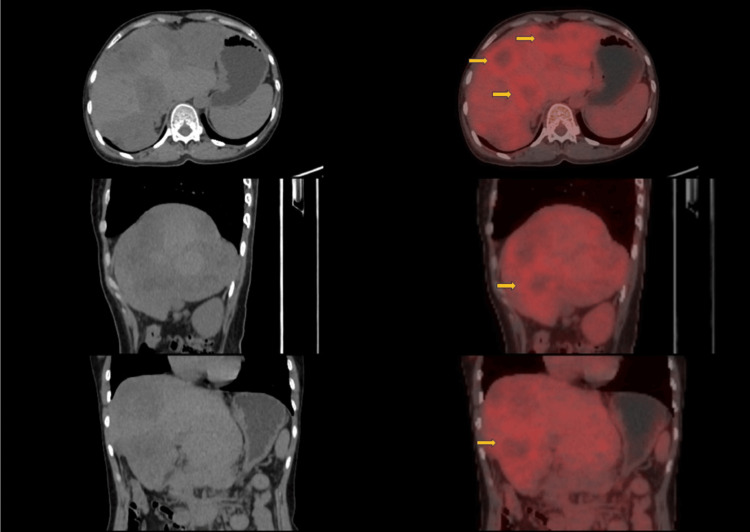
18F-FDG PET-CT image of the abdomen The PET image shows the axial (top row) and coronal (middle and bottom rows) sections. The left panel demonstrates contrast-enhanced CT images, while the corresponding right panel shows fused PET-CT images. There are multiple, heterogeneously enhancing hepatic lesions involving both lobes, predominantly the right lobe of the liver, with intense and heterogeneous FDG uptake within the lesion (yellow arrows), consistent with a metabolically active tumor. Background liver parenchyma shows comparatively lower physiological uptake. FDG: fluorodeoxyglucose, PET-CT: positron emission tomography-computed tomography.
